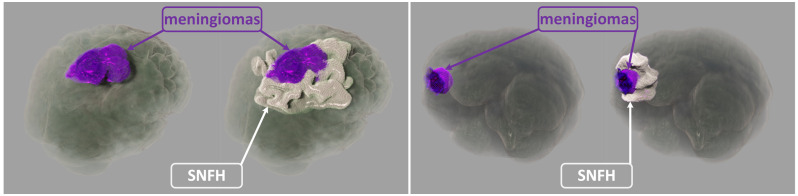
The visualization of meningiomas (encompassing both enhancing tumor and non-enhancing tumor core regions) and the surrounding non-enhancing T2/FLAIR hyperintensity (SNFH) from BraTS 2023 dataset [21] using the SegR3D system.
